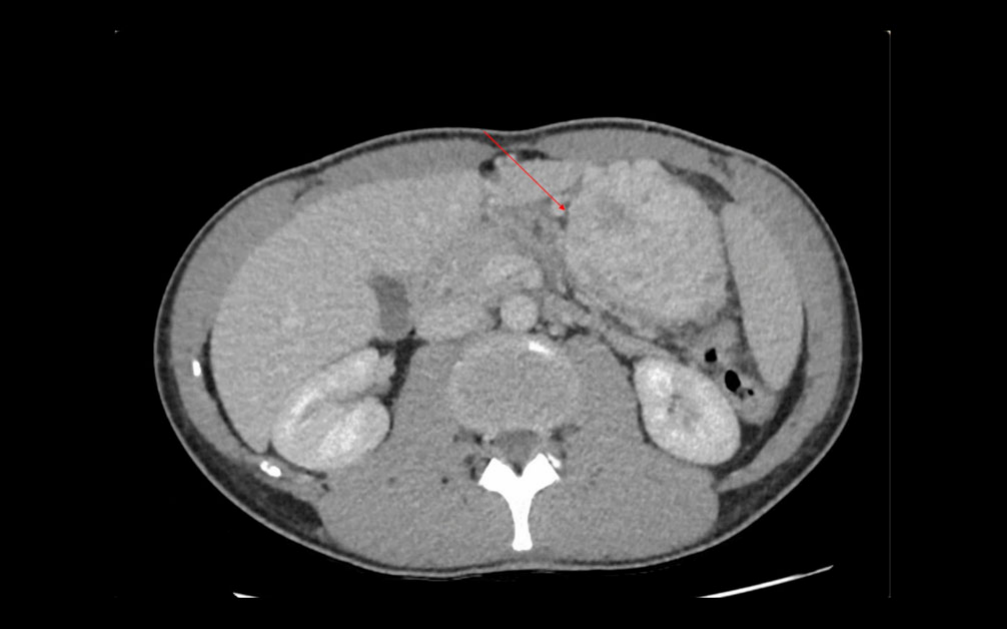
Computed tomography scan showing the intra‐abdominal lesion.
